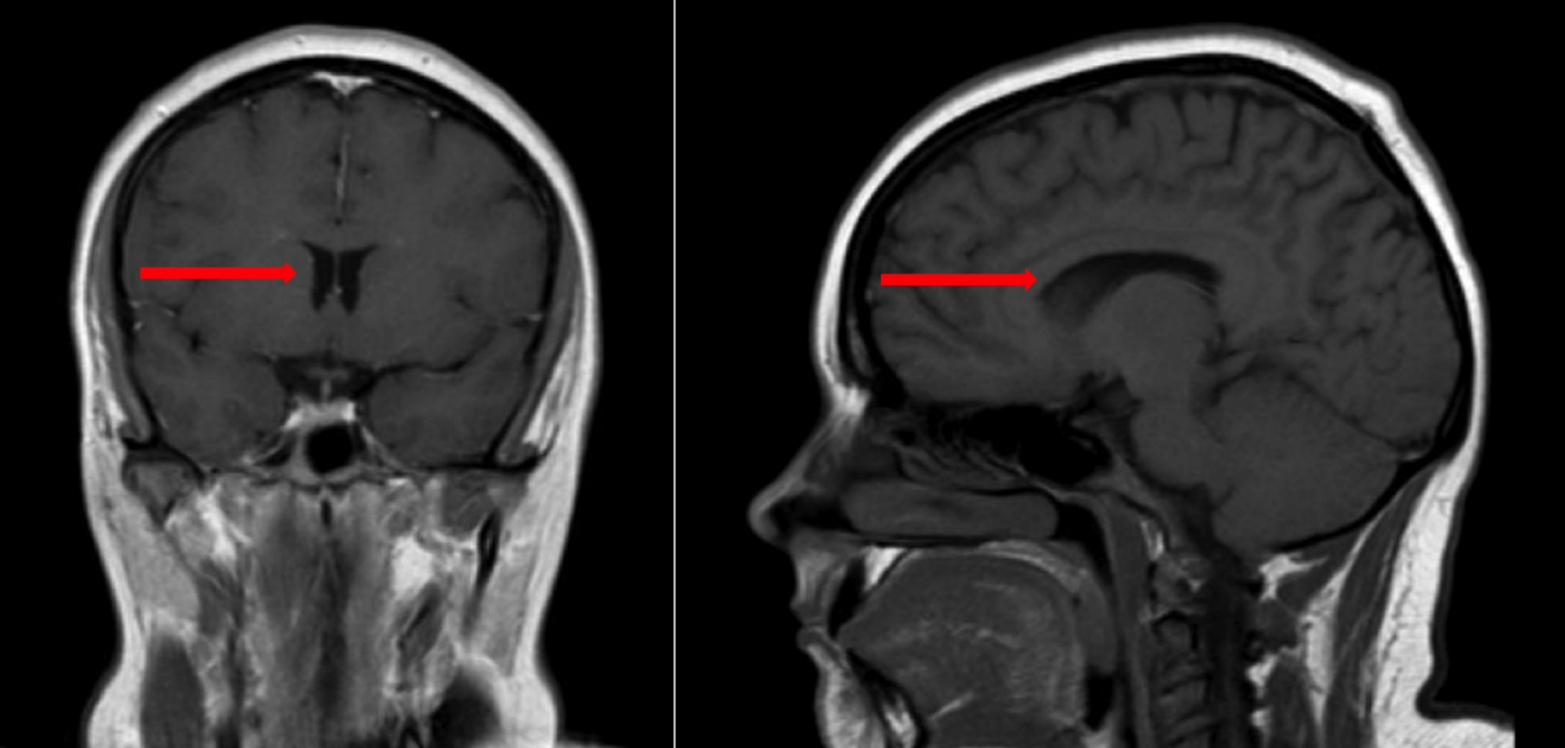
T1-weighted MRI of the Brain Sections from a normal T1-weighted magnetic resonance imaging (MRI) of the patient's brain. These images show no abnormalities that would result in secondary intracranial hypertension. Red arrows point to a normal ventricular system.
